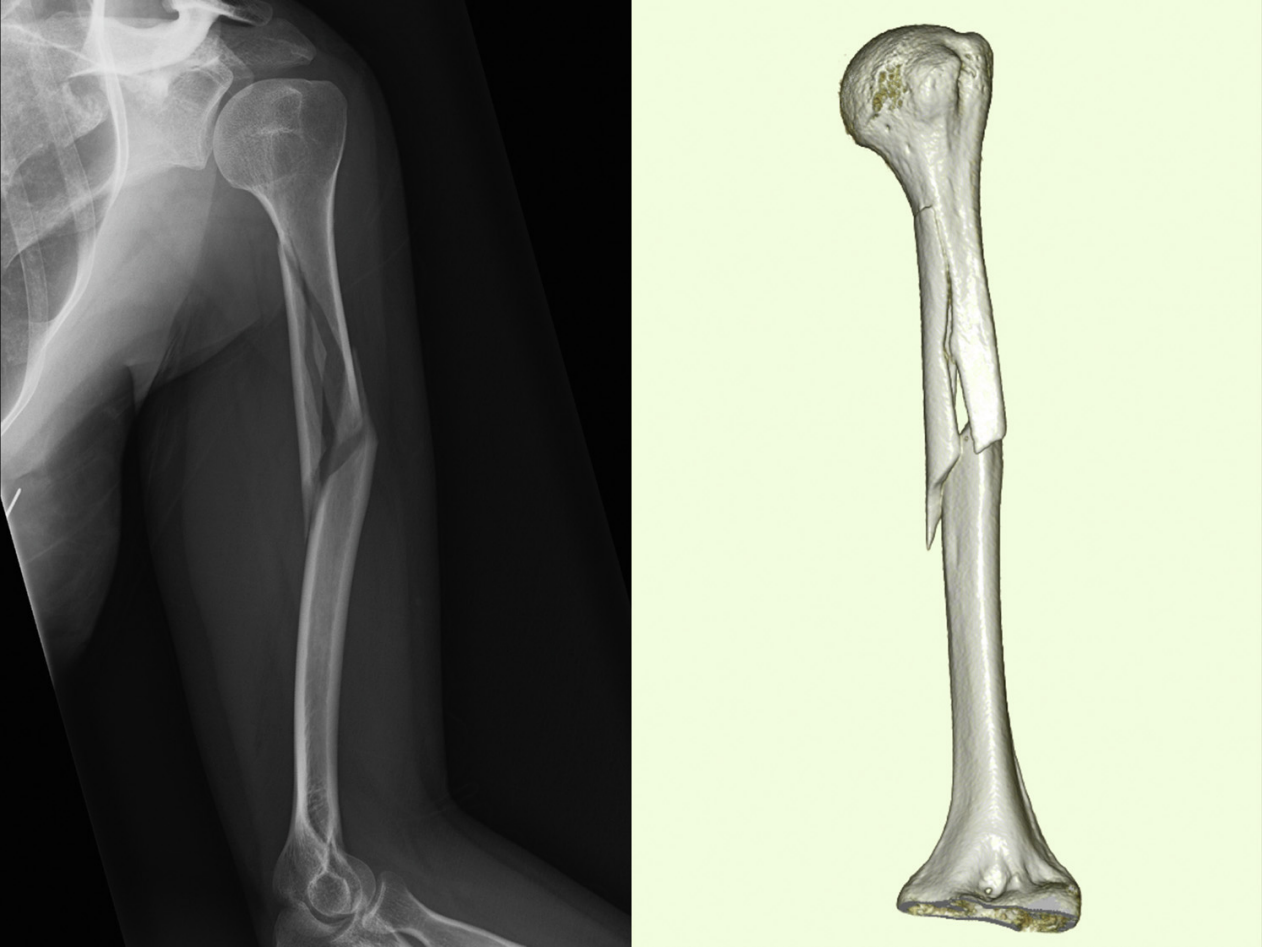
Plain radiographs and CT scans showing the oblique multifragmentary fracture of the left humeral shaft. *CT*, computed tomography.
